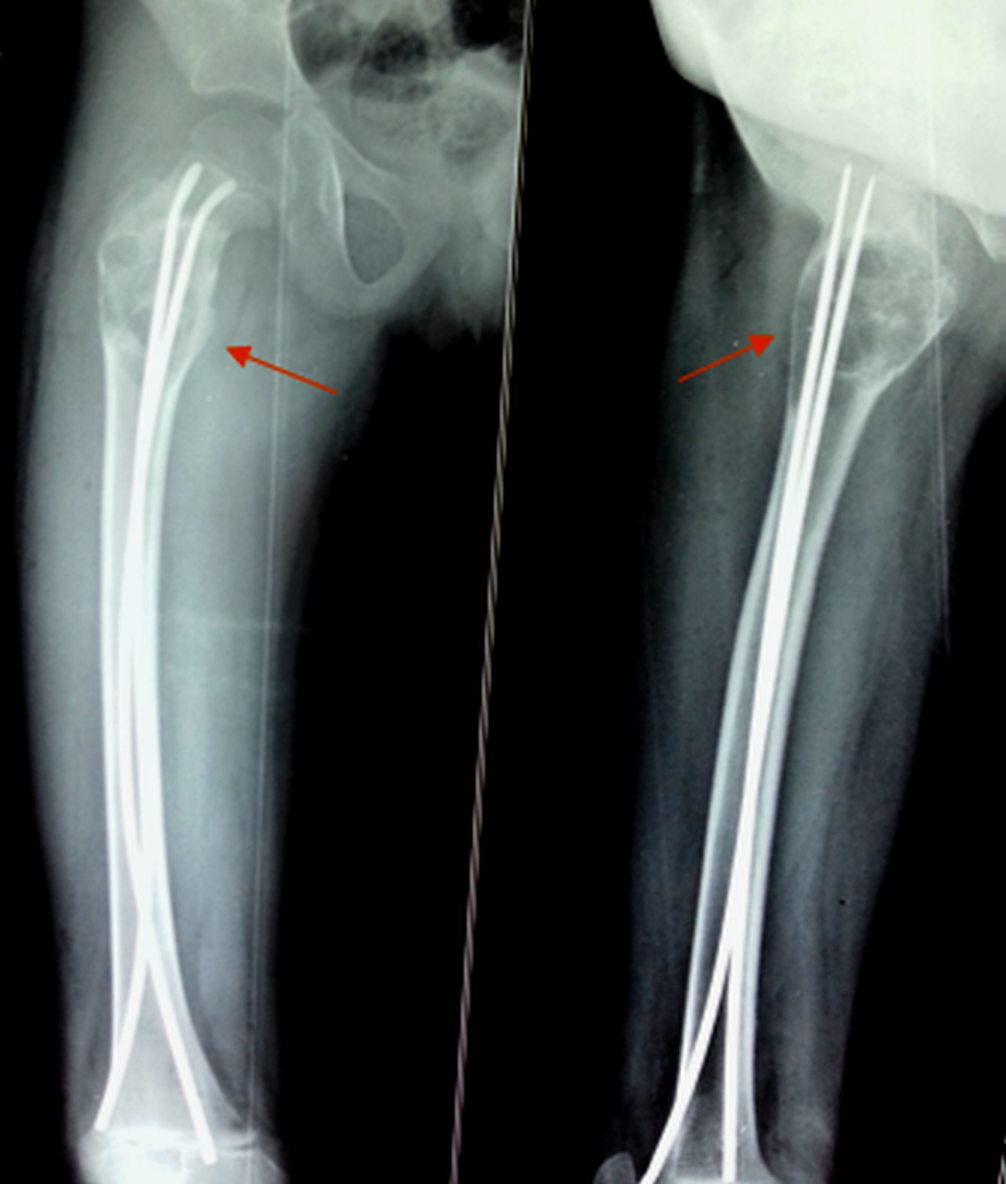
Postoperative radiograph (anteroposterior and lateral views) Shows fixation with ender’s nails after injecting a sclerosing agent in the lesion.
